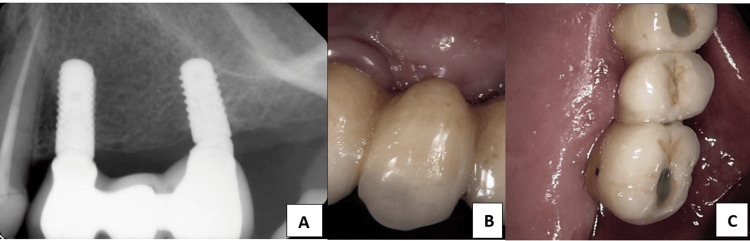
(A) Periapical X-ray showing the two implants restored and splinted together with a fixed partial denture (fixed prosthesis). (B, C) Clinical photos taken with an intraoral camera (Dentsply Sirona’s intraoral scanner (York, USA)) for the fixed partial denture.
